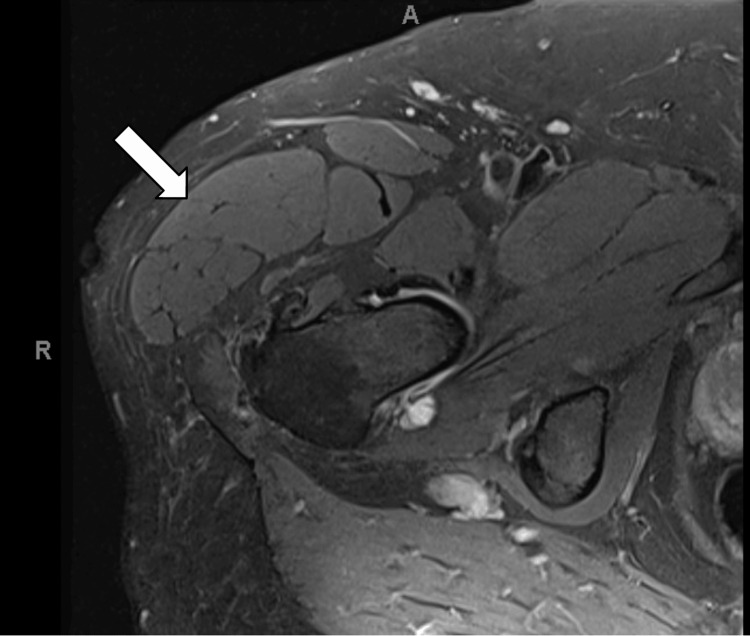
MRI showing a cross-section of TFL hypertrophy The scan of the right lower extremity at the level of the hip in the axial plane shows the enlargement of the TFL muscle (white arrow). TFL, tensor fascia lata; MRI, magnetic resonance imaging
